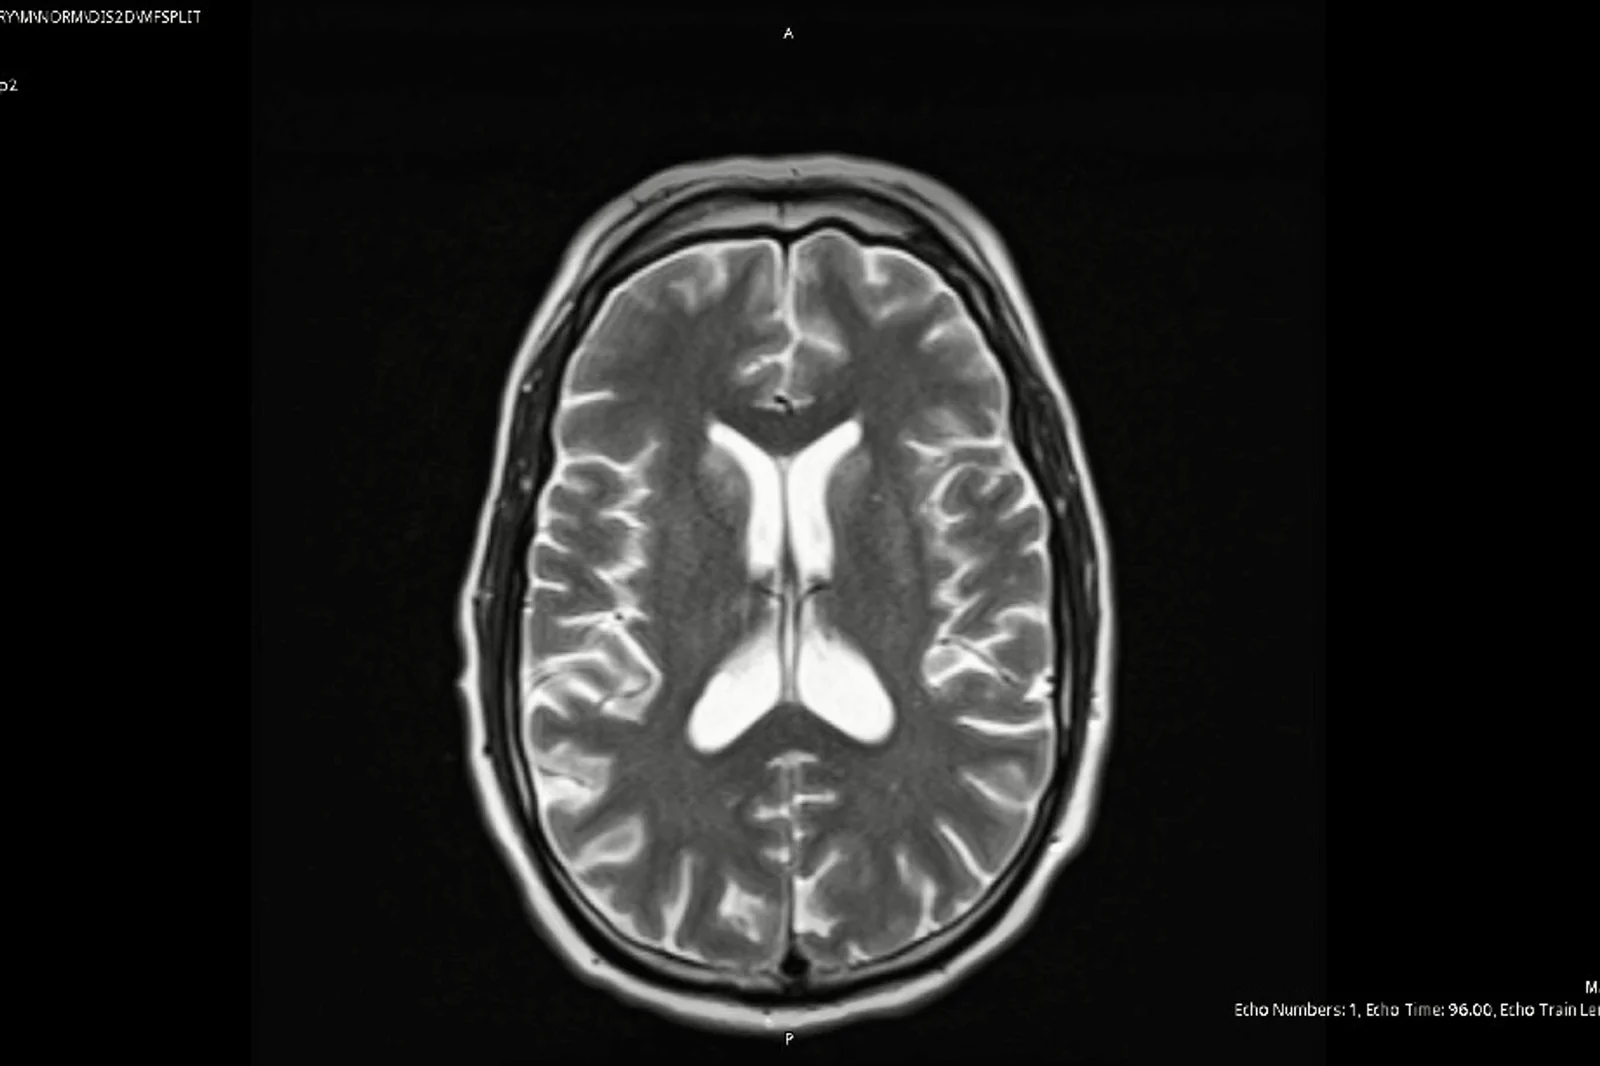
MRI of the brain is normal with no radiologic evidence of metastasis or encephalitis
